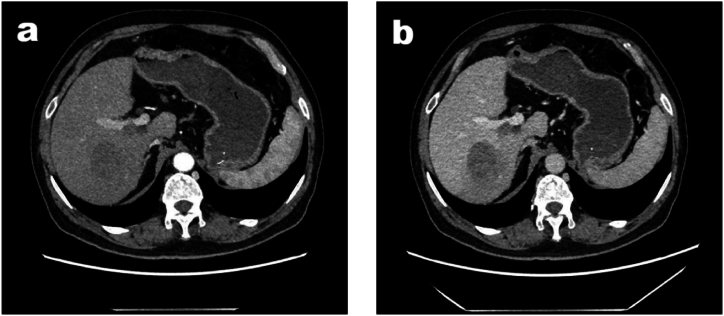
(a). Enhanced CT (arterial phase) showed that the tumor was located in the right posterior lobe with uneven enhancement. Fig. 1b. Enhanced CT (venous phase) showed that the tumor was further enhanced in the venous phase, and the right branch of the portal vein was invaded.
